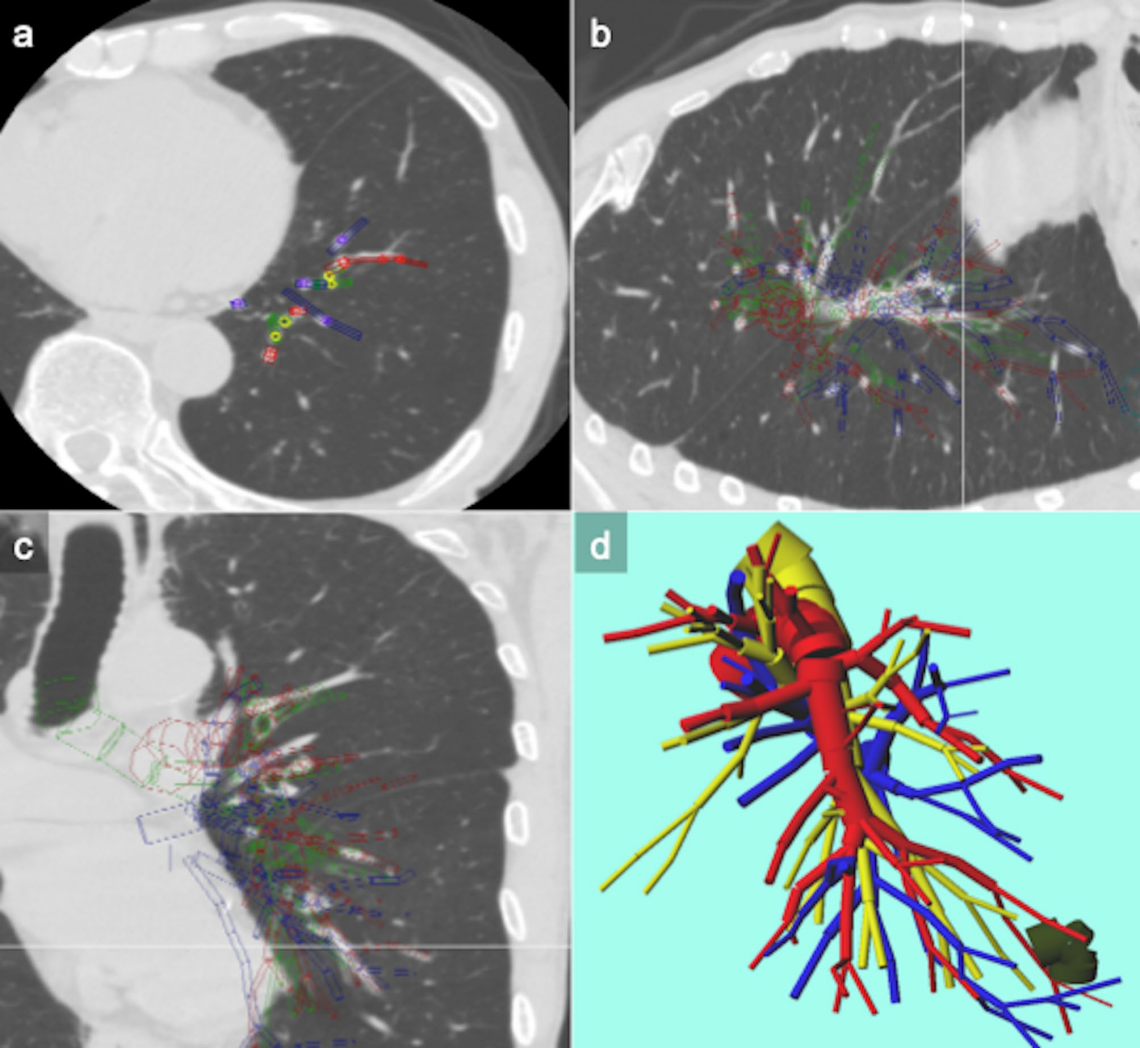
Creation of patient-actual virtual 3D pulmonary model After the communications-in-medicine (DICOM) format images, which were obtained from 1 mm thin-sliced high-resolution computed tomography (HRCT)-scan images of tumor and hilum, were uploaded to a personal computer, the homemade software “CTTRY” (Tokyo Women’s Medical University, Tokyo, Japan) allowed surgeons to mark pulmonary arteries, veins, bronchi, and tumor on the HRCT image manually and attempt to reconstruct an anatomical model with the help of anatomically correct images (A–C). The location and thickness of the pulmonary vessels and bronchi were rendered as various sizes of cylinders. In accordance with the resulting numerical data, a 3D image was reconstructed with Metasequoia shareware (http://metaseq.net/) (D)
